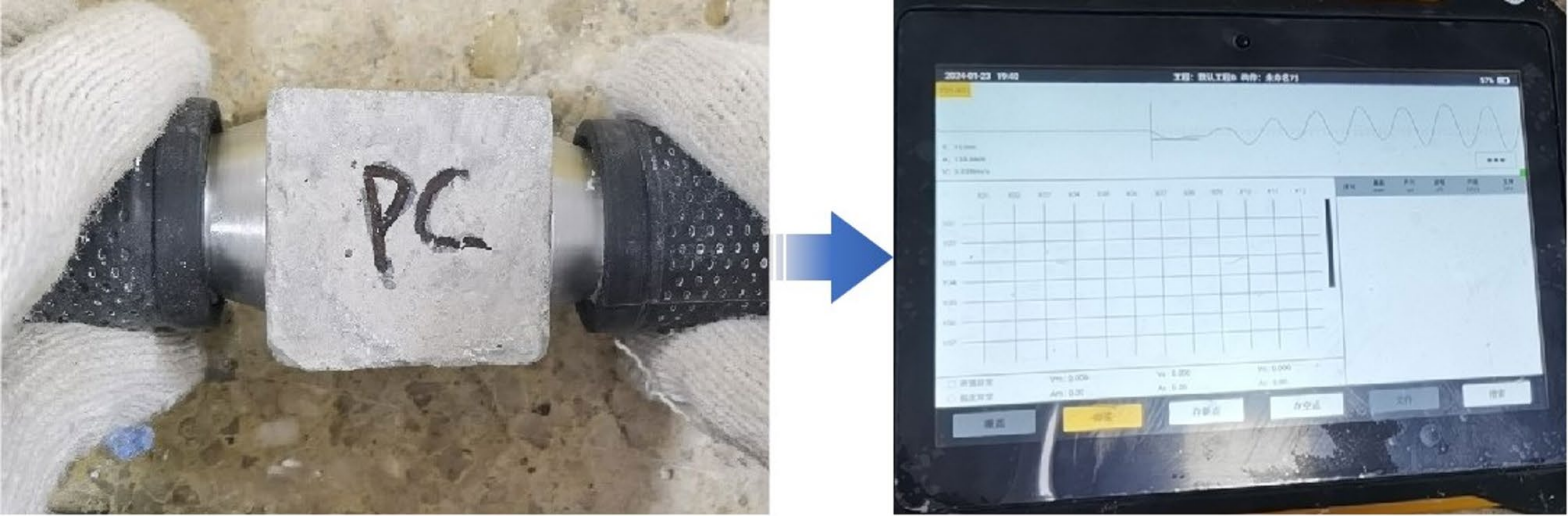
Ultrasonic testing instrument test chart.
